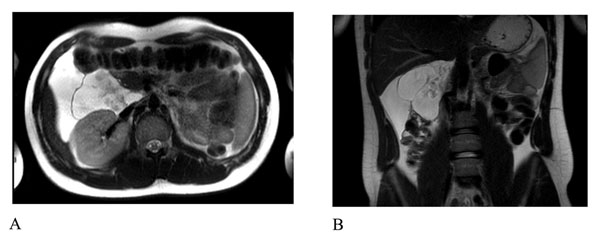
MRI of the abdomen. Axial (a) and coronal (b) T2-weighted TSE images showed the presence of a huge fluid-filled mass with internal septa located in the hepato-renal space extending from the hepatic hilum to the right colon and displacing medially the second duodenal segment and anteriorly the hepatic flexure of the colon.
